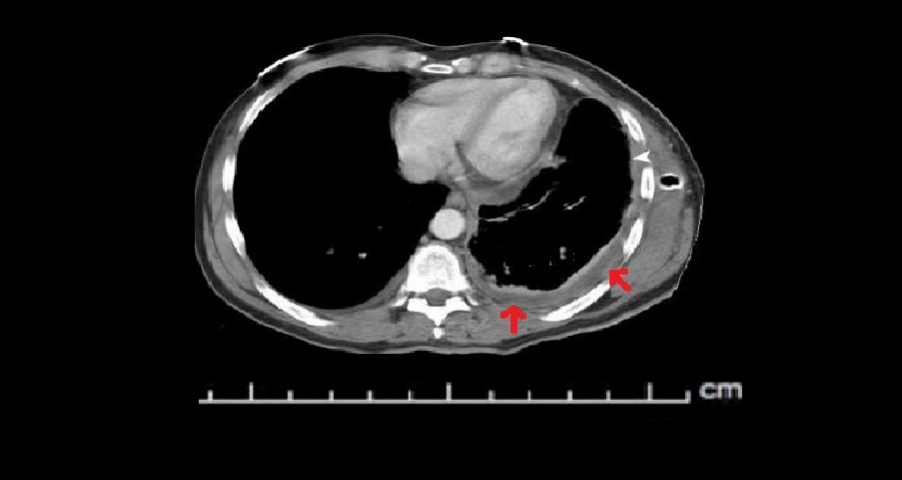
A computed tomography angiogram (CTA) showing mild left pleural effusion (red arrows) and a small pericardial effusion
